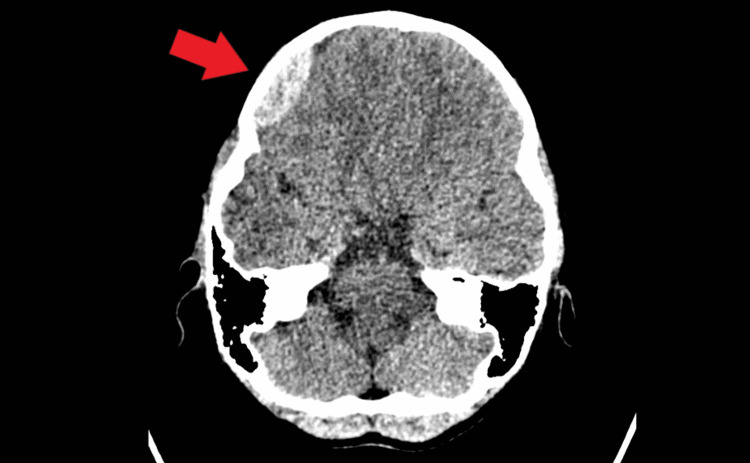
The red arrow shows a convex-shaped hyperdensity over the right frontal region indicating a right extra-axial hematoma on a non-contrast computed tomography of the brain.
